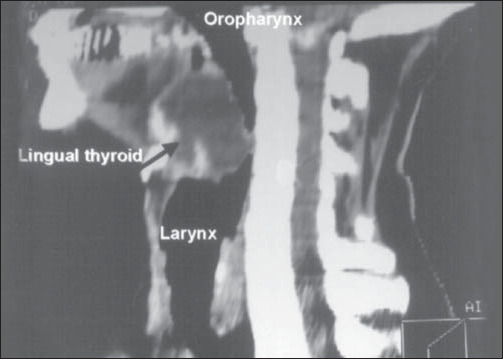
Computed tomography imaging scan demonstrating an oval-shaped mass at the base of the tongue causing sub-occlusion in the oropharynx.
